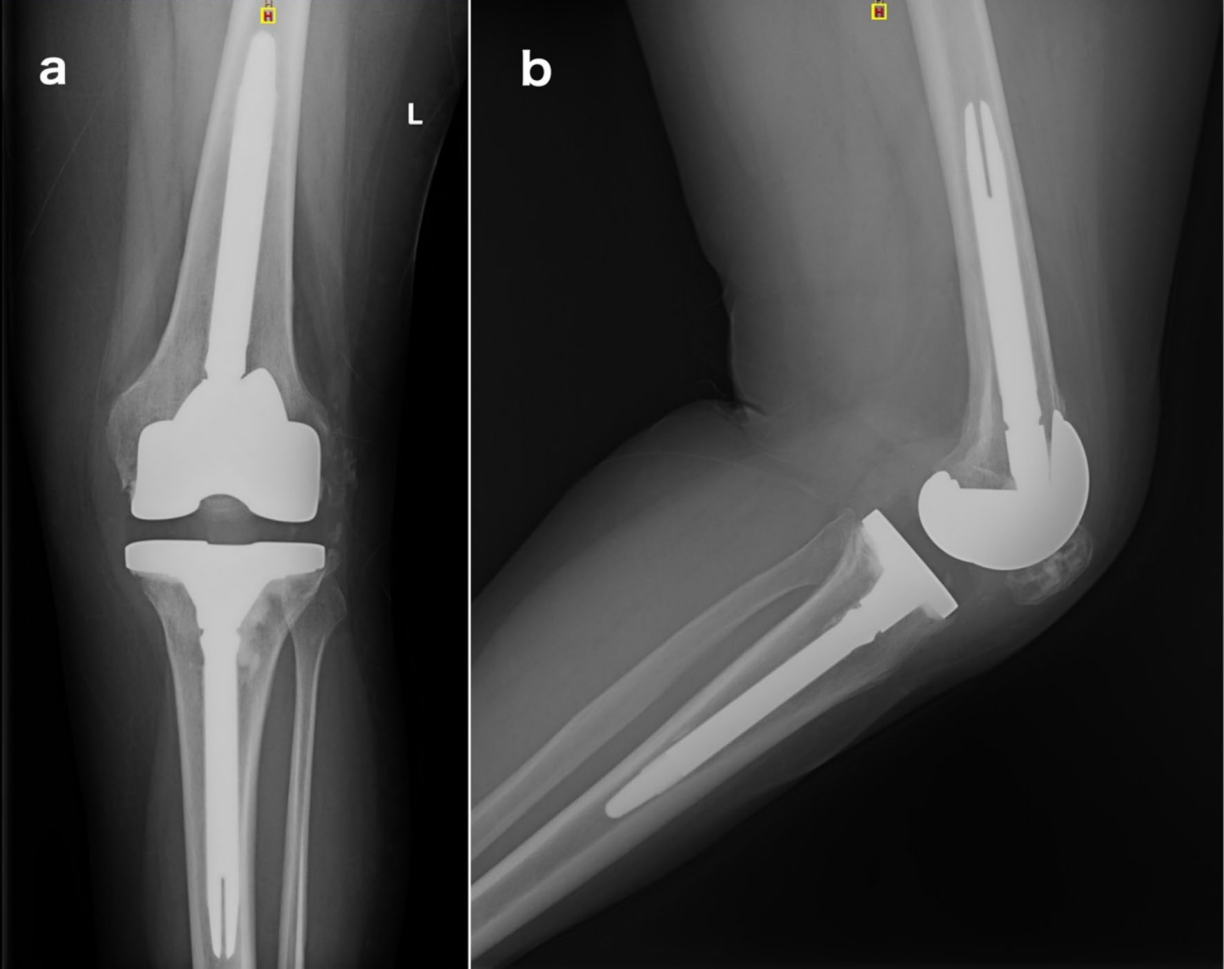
a Anteroposterior and b lateral pre-operative radiography of an infected (sinus tract) revision prosthesis due to Staphylococcus epidermidis
